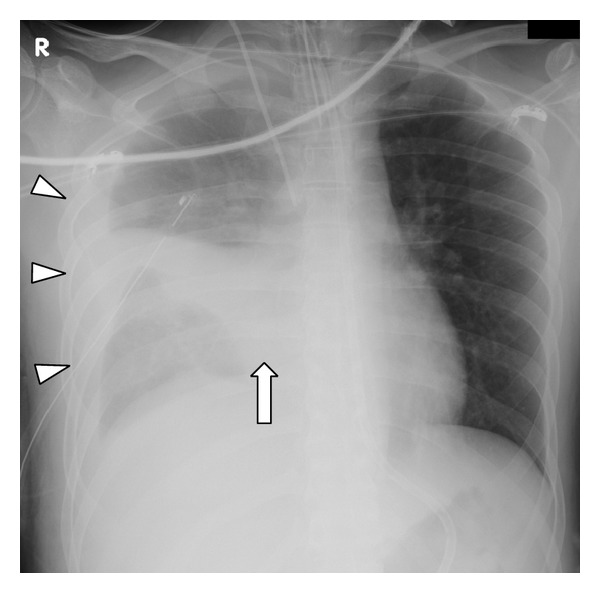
Chest radiograph obtained on the fourth day after admission shows right middle lobe atelectasis (arrow) and right-sided hemothorax (arrowhead).
